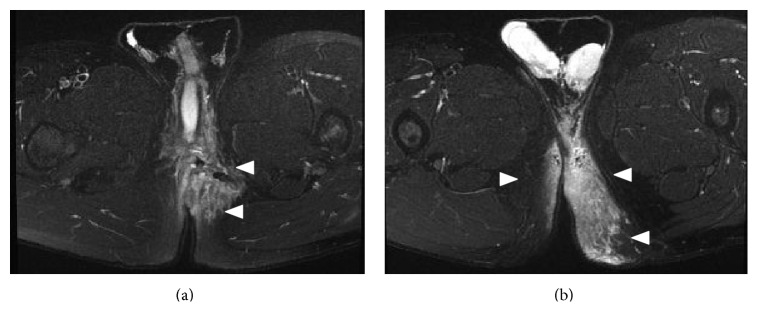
STIR sequence of the MRI showed high intensity around the perineum.
